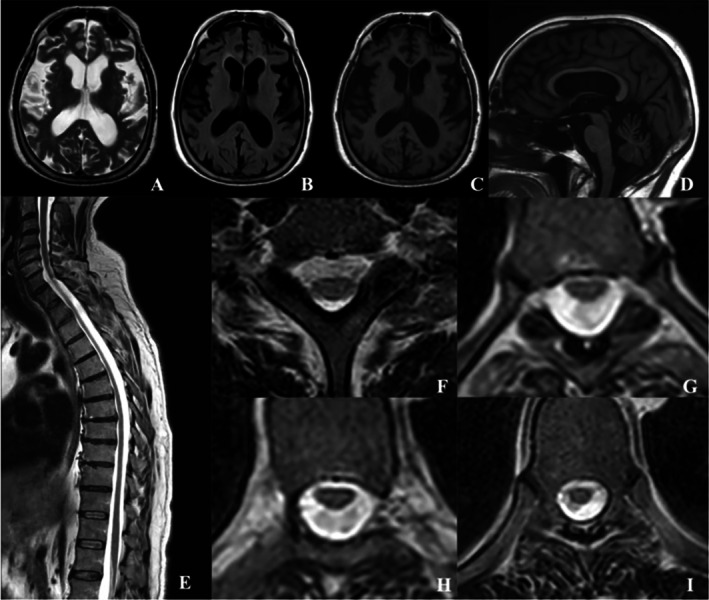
Neuroimaging assessment. Brain and spine MRIs. Family ITA201—Patient II:1. Neuroimaging findings of dementia. Axial spin‐echo (SE) T2‐weighted (A), axial Fluid Attenuated Inversion Recovery (FLAIR) T2‐weighted (B), and axial SE T1‐weighted (C) show severe atrophy of the temporal lobes and enlargement of the Sylvian fissures. Index Brazilian Patient with intellectual disability. (D) Midline sagittal SE T1‐weighted MRI scan showing generalized thinning of the *corpus callosum*, without focal lesions. Index Japanese Patient. MRI of the spine. (E) Sagittal Turbo SE (TSE) T2‐weighted shows widespread thinning of the spinal cord. Axial TSE T2‐weighted at C7 (F), D5 (G), D7 (H), and D9 (I) level confirmed the reduction in thickness of the spinal cord without abnormal medullary signal intensity.
